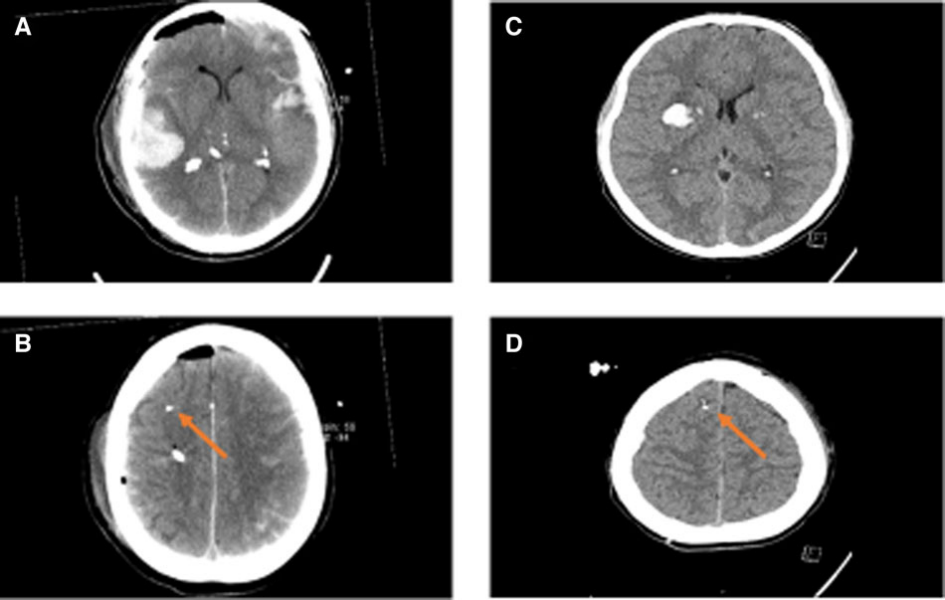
Computed tomography scans of case nos. 1 and 9—structural injuries and relation to the CMD catheter. The figure demonstrates the computed tomography scans of cases no. 1 (A,B) and no. 9 (C,D). Case no. 1 exhibited extensive mixed lesions of particularly cerebral contusions and traumatic subarachnoid hemorrhage (A). The cerebral microdialysis catheter (red arrow) was located in the injured lobe, but in the normal-appearing brain, distant from any hemorrhagic lesion (B). Case no. 9 exhibited a deep cerebral contusion (C). The CMD catheter (red arrow) was also located in the injured lobe, but in the normal-appearing brain, distant from any hemorrhagic lesion (D). CMD, cerebral microdialysis.
